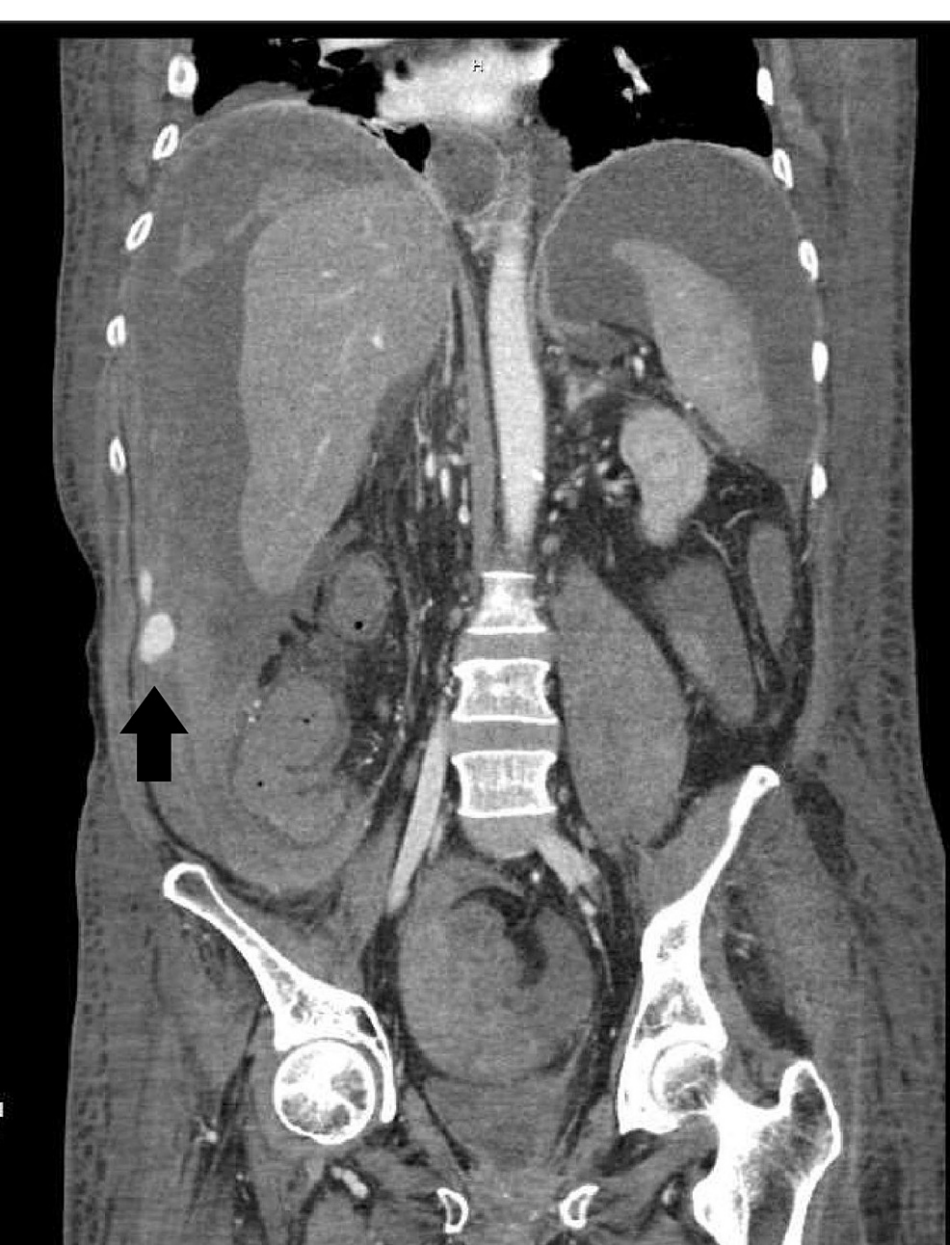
CT with angiography showing hemoperitoneum with hemorrhagic varix (black arrow); coronal view.
